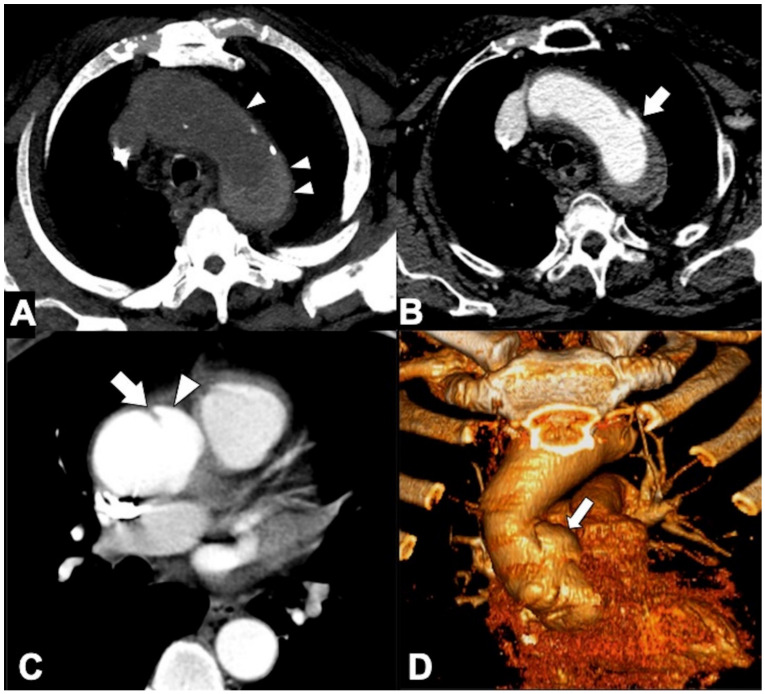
CTA features of Svensson’s Class 4 (PAU) and Class 3 (limited intimal tear) variants. (A) Axial unenhanced CT image shows aortic arch high-density intramural hematoma (IMH) in a 74-year-old male with acute neck and chest pain (arrowheads). (B) CTA axial scan in the same patient shows a flat penetrating ulcer (arrow) with associated IMH in the arch convexity. (C) CTA axial image shows a linear filling defect with subtle undermined edges (arrow) and eccentric medial one-sided bulge of the ascending aorta (arrowhead) without a clear intimomeadial flap or false lumen in a 58-year-old male with sudden onset of chest and back pain. (D) 3D-VR oblique coronal reconstruction confirms the eccentric one-sided saccular aortic bulging (‘‘mushroom cap’’ morphology) of class 3 dissection (arrow).
